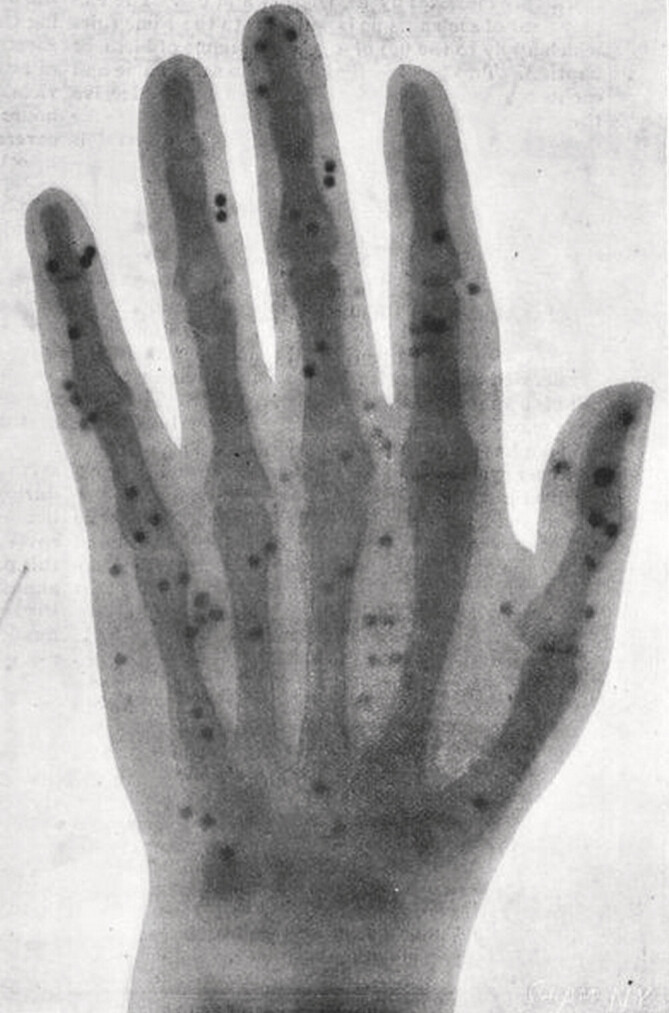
Print of the X-ray of Prescott Hall Butler’s left hand containing about 72 pellets, taken by M.I.Pupin at Columbia College, New York, on February 19,1896. Dr. Pupin’s X-ray photograph of a wounded hand. Scientific American, 21 March, 1896: LXXIV(12):184) [rerif]
